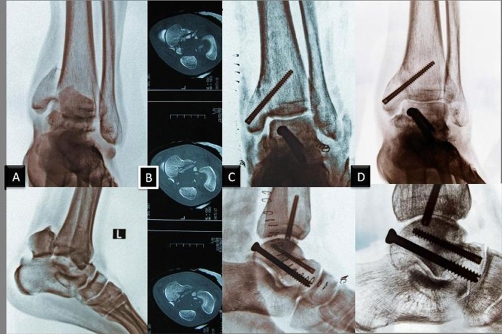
Preoperative and postoperative radiological data's of Case 1. Preoperative anteroposterior and lateral plain radiographs (A). Computerized tomography scans demonstrated posterior fragment displacement with a degree of comminution of the center of the talar dome (B). Postoperative anteroposterior and lateral plain radiographs; the talus body fractures and the fractures of the medial malleolus were fixed through anteromedial approach using AO cannulated and Acutrak^®^ headless screws and additional screws were inserted from posterior to anterior percutaneously. Because posteroanterior screw position gave more stability (C). Anteroposterior and lateral plain radiographs following 16 months of follow-up (D).
